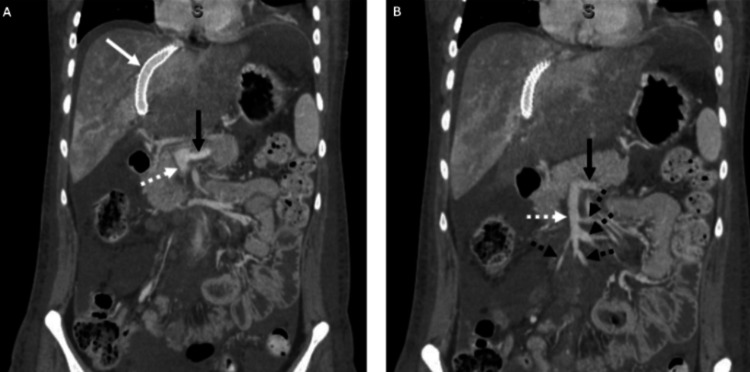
Post-procedure abdominal CT. (A) Coronal CT demonstrating patent TIPS (solid white arrow), patent distal splenic vein (solid black arrow), and superior mesenteric veins (dotted white arrow). (B) Coronal CT demonstrating patent distal splenic vein (solid black arrow), superior mesenteric vein (dotted white arrow), and multiple patent branches of the superior mesenteric vein (dotted black arrow). TIPS, transjugular intrahepatic portosystemic shunt
